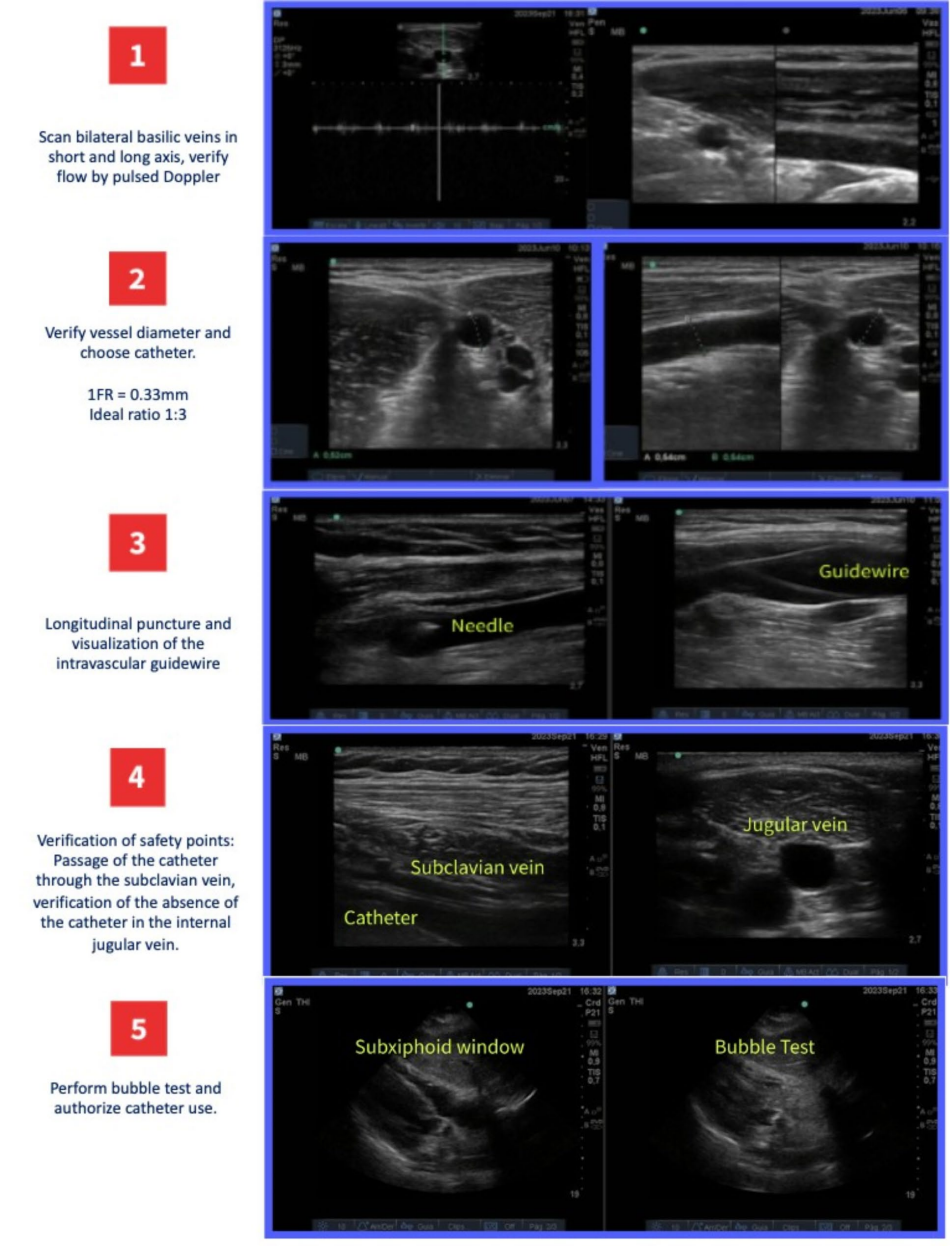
Steps for the insertion of a peripherally inserted central venous catheter using the ultrasonographic technique
